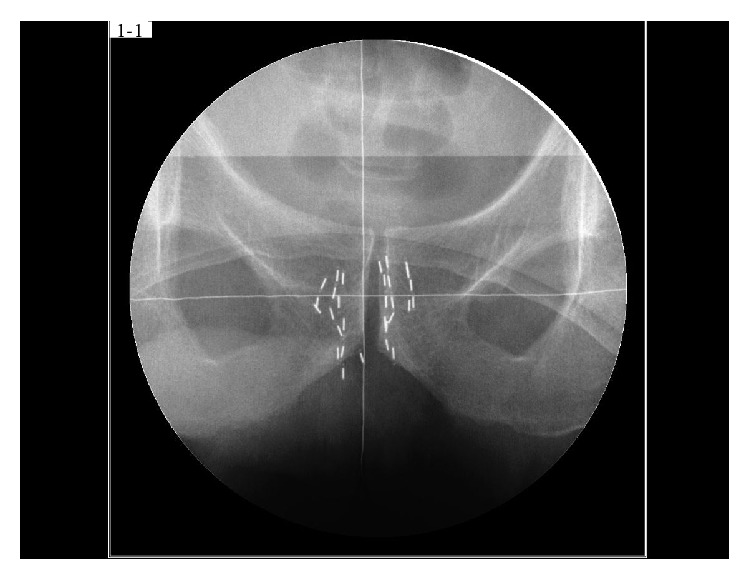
Pelvic X-ray image after salvage brachytherapy.
